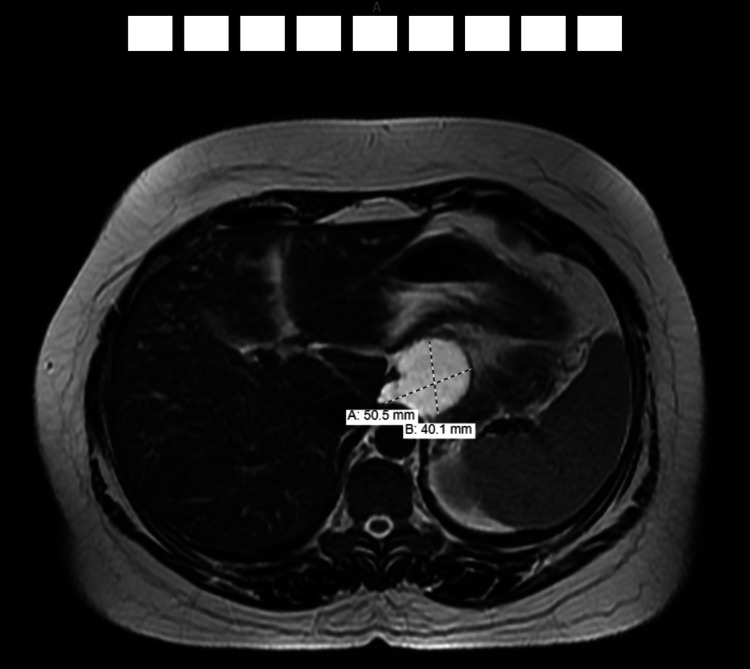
MRI findings MRI confirms the presence of a well-marginated 5 cm cyst in the left upper abdomen, likely arising from the right diaphragmatic crus as described above. It seems to be displacing the pancreas rather than arising from it. Again, differential diagnosis includes a foregut duplication cyst or a bronchogenic cyst, among others MRI: magnetic resonance imaging
